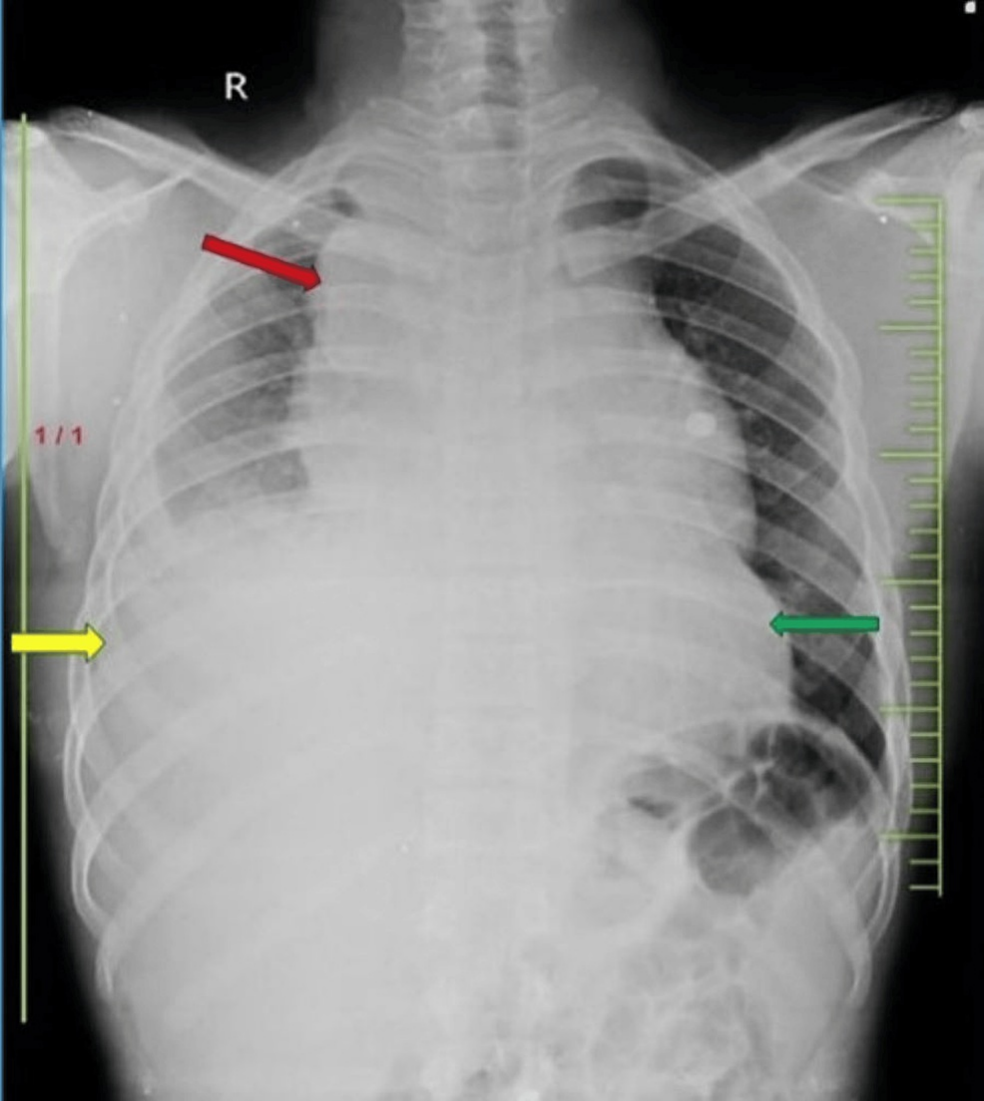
Chest X-ray showing a mediastinal mass in the right upper and middle zone extending above the clavicle (red arrow), right-sided pleural effusion obscuring the right costophrenic angle (yellow arrow), and mild cardiomegaly (green arrow).
